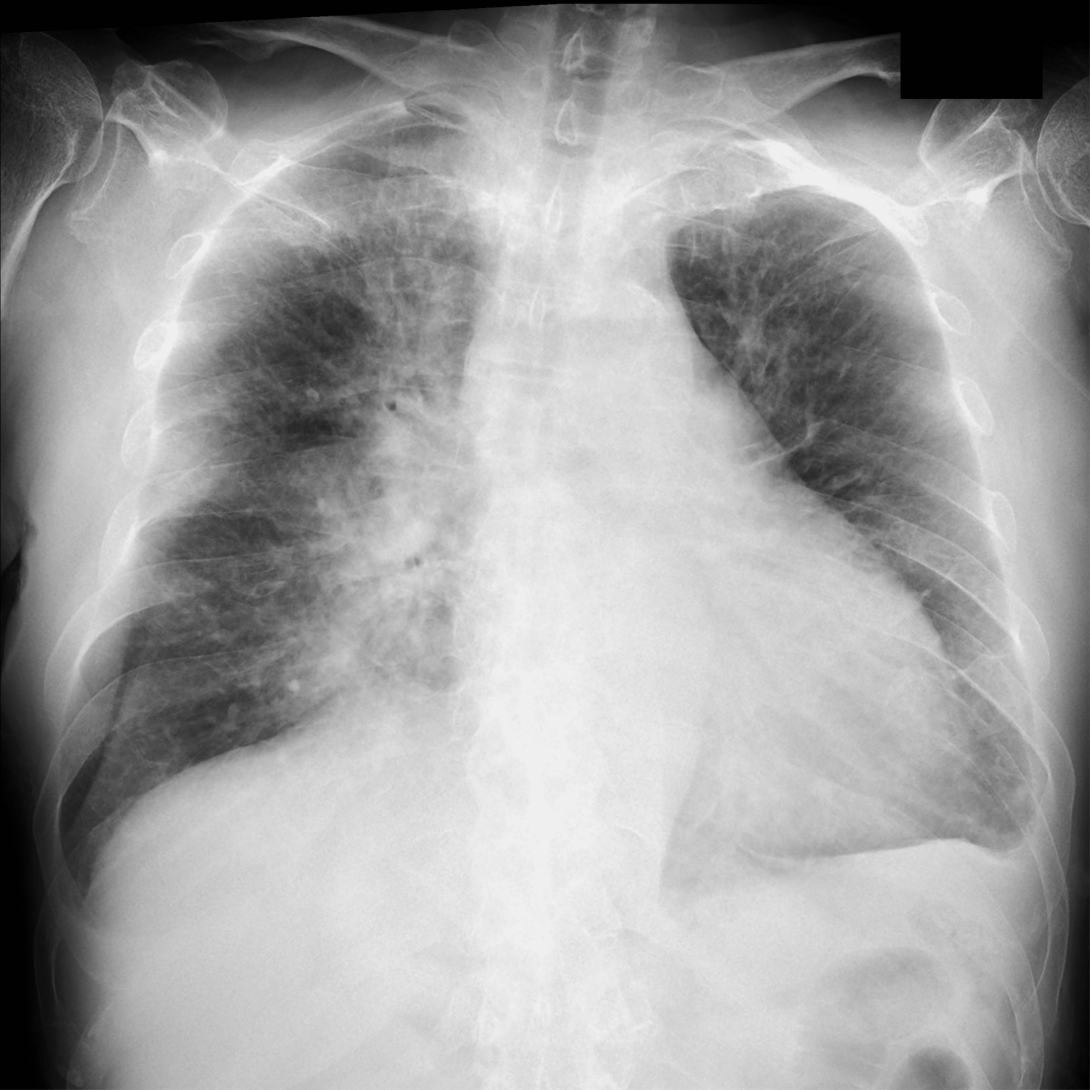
Chest X-ray image Cardiomegaly and marked pulmonary congestion are visible in both lung fields.
